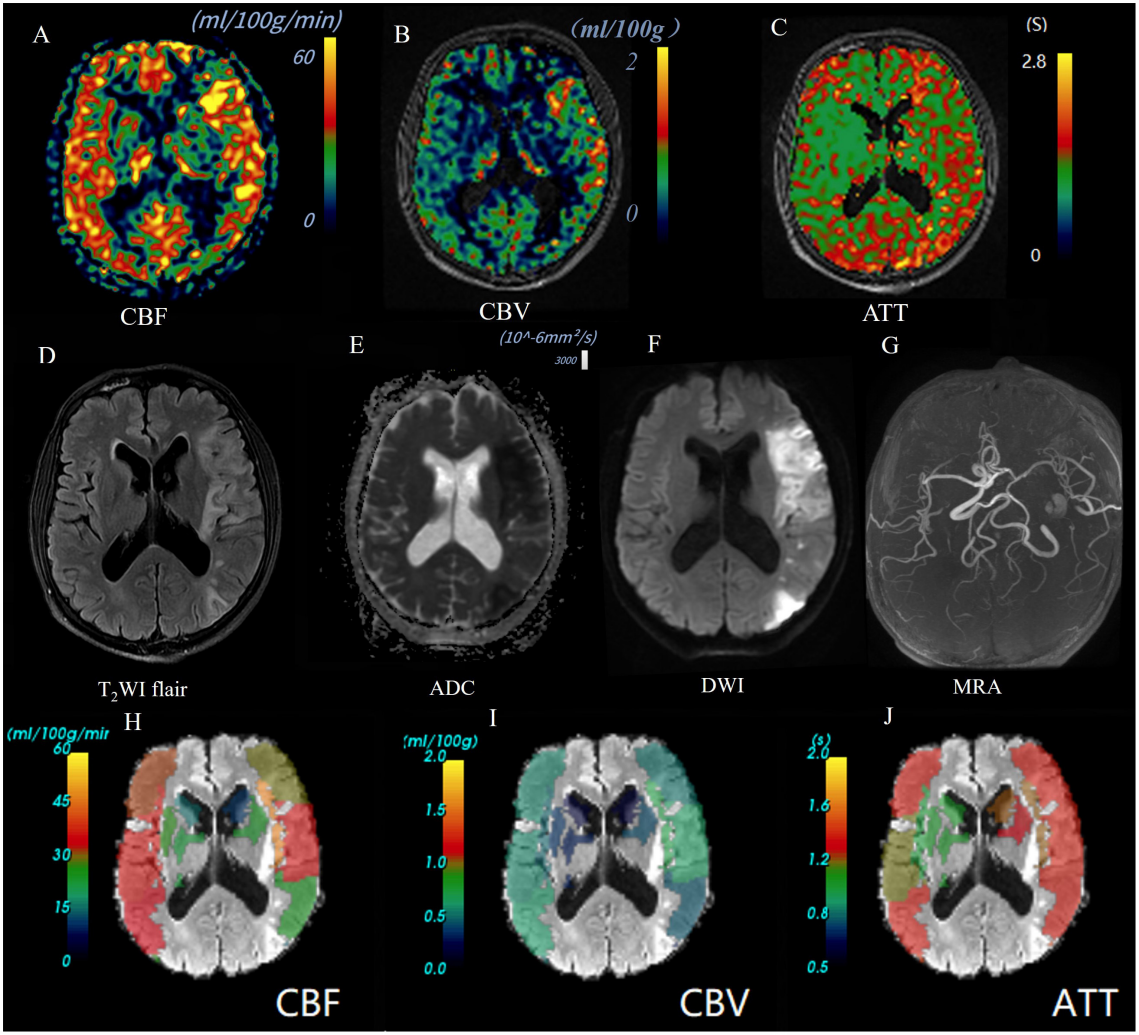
Images of an AIS patient with a poor outcome (90-day mRS = 4). A 55-year-old male patient was diagnosed as AIS in left temporal parietal occipital lobe. Baseline NIHSS score 10 points, 90 d mRS score 4 points. CBF perfusion map (A) and CBV perfusion map (B) showed high perfusion of the lesion; ATT perfusion map revealed extended arrival time (C). The lesion showed high signal on T2Flair (D) and DWI map (b = 1,000) (F), and the ADC value decreased (E). 3D-TOF-MRA map showed the left middle cerebral artery is narrow but not occluded (G). Warm-to-cold color bars represent values from high to low. The ASPECTS template was used to standardize the perfusion parameters across 10 different zones. (H–J) The scores obtained were as follows: CBF-ASPECTS: 6 points, CBV ASPECTS: 9 point, ATT-ASPECTS: 8 points.
